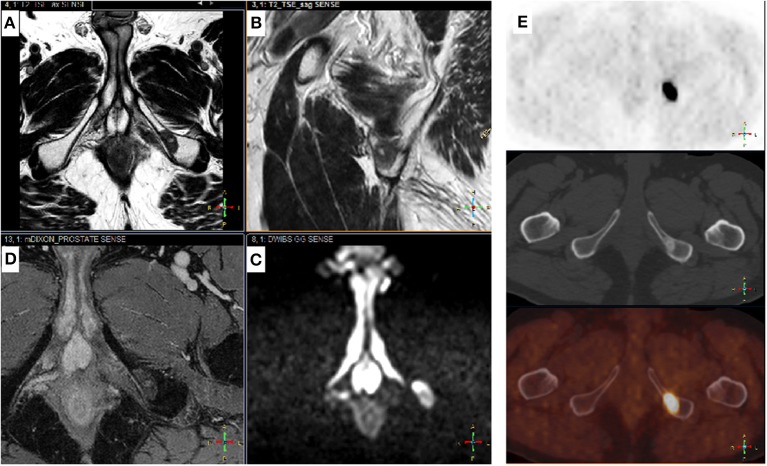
Complementary Choline-TEP and pelvic MRI. Patient with a history of Gleason 7 (4 + 3) prostatic adenocarcinoma treated by radical prostatectomy 9 years ago (pT3aN0M0R1). PSA recurrence 5 years later followed by EBRT, external beam radiation therapy. Three years later, rising PSA from 0.5 to 1.4 ng/mL in 6 months. Prostate MRI performed before 18-F choline-PET-CT shows no sign of recurrence in the prostatectomy bed, but detected a 17-mm suspicious bone lesion in the left ischiopubic branch. Typical signal on axial (A) and sagittal (B) T2-w images were low and homogeneous; high on b-1000 axial diffusion-weighted images (DWI) (C) and high on late T1-w dynamic contrast-enhanced (DCE) images (D), corresponding to high cellular density and hypervascularization, respectively. 18F choline-PET-CT (E): axial slices of the pelvis showing an osteosclerotic lesion on the right ischiopubic branch with high uptake of the tracer. From top to bottom: PET, CT, PET-CT.
